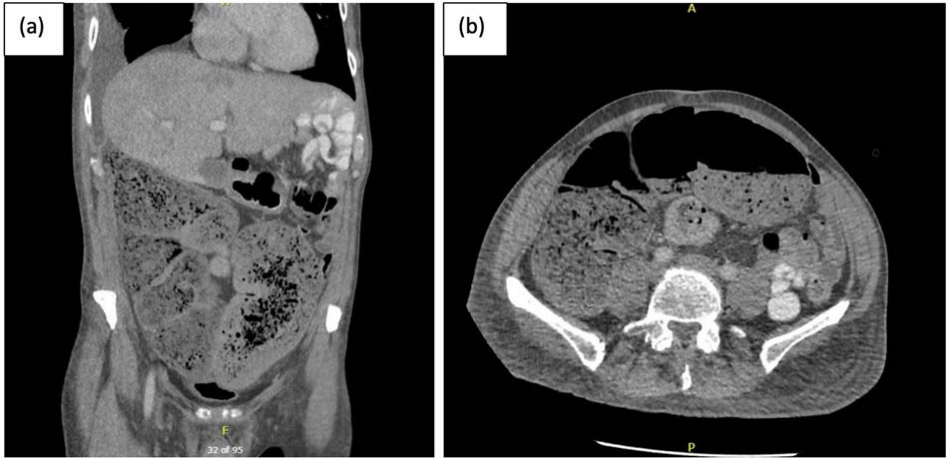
(a) Coronal and (b) axial views of CT abdominal pelvis, with oral and intravenous contrast demonstrating significant faecal loading in ascending colon and proximal to mid-transverse colon.
